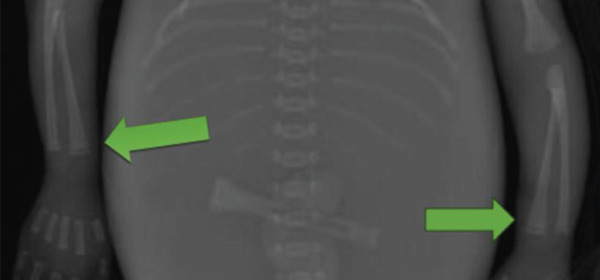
Radiographic lucent bands of the long bones.
